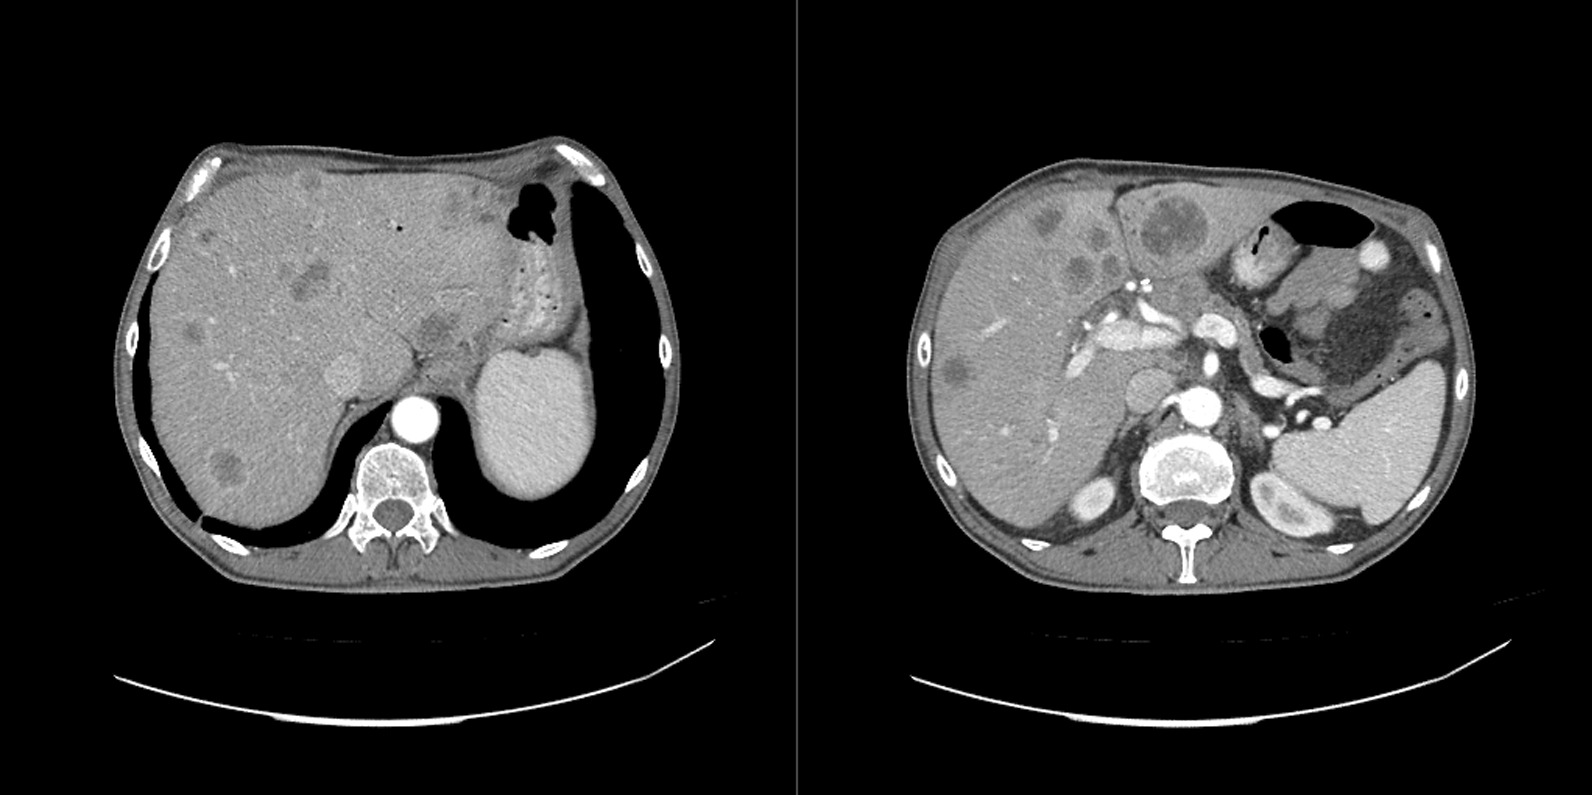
Magnetic resonance imaging image of the liver metastatic lesions. Patient 2
